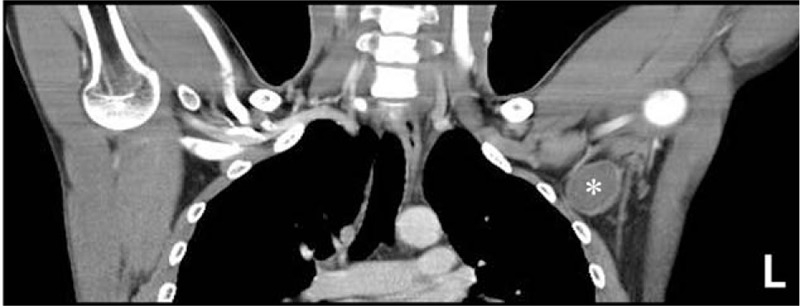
Preoperative CT. A well-demarcated mass of approximately 3.5 × 3.4 cm, with features consistent with those of a fibroadenoma, was observed at low attenuation in the left axillary region, denoted as an asterisk mark (^∗^). CT = computed tomography.
